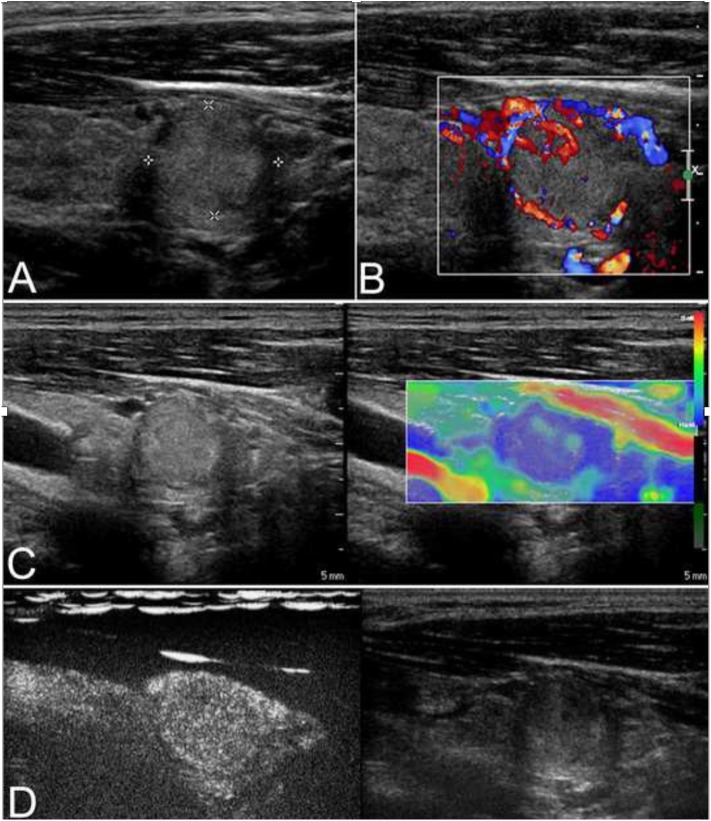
This case is a 27-year-old man with an follicular carcinoma (FC) nodule. (A) Conventional ultrasonography (CUS) showed a solid hyperechoic nodule in the left lobe that was associated with a regular thin halo and an inside area that was hypoechoic, which is indicative of low suspicion. (B) Abundant intranodular and peripheral vascularity was detected. (C) The elastography score was 2, indicating a soft stiffness. (D) Contrast-enhanced ultrasonography (CEUS) revealed heterogenous enhancement.
